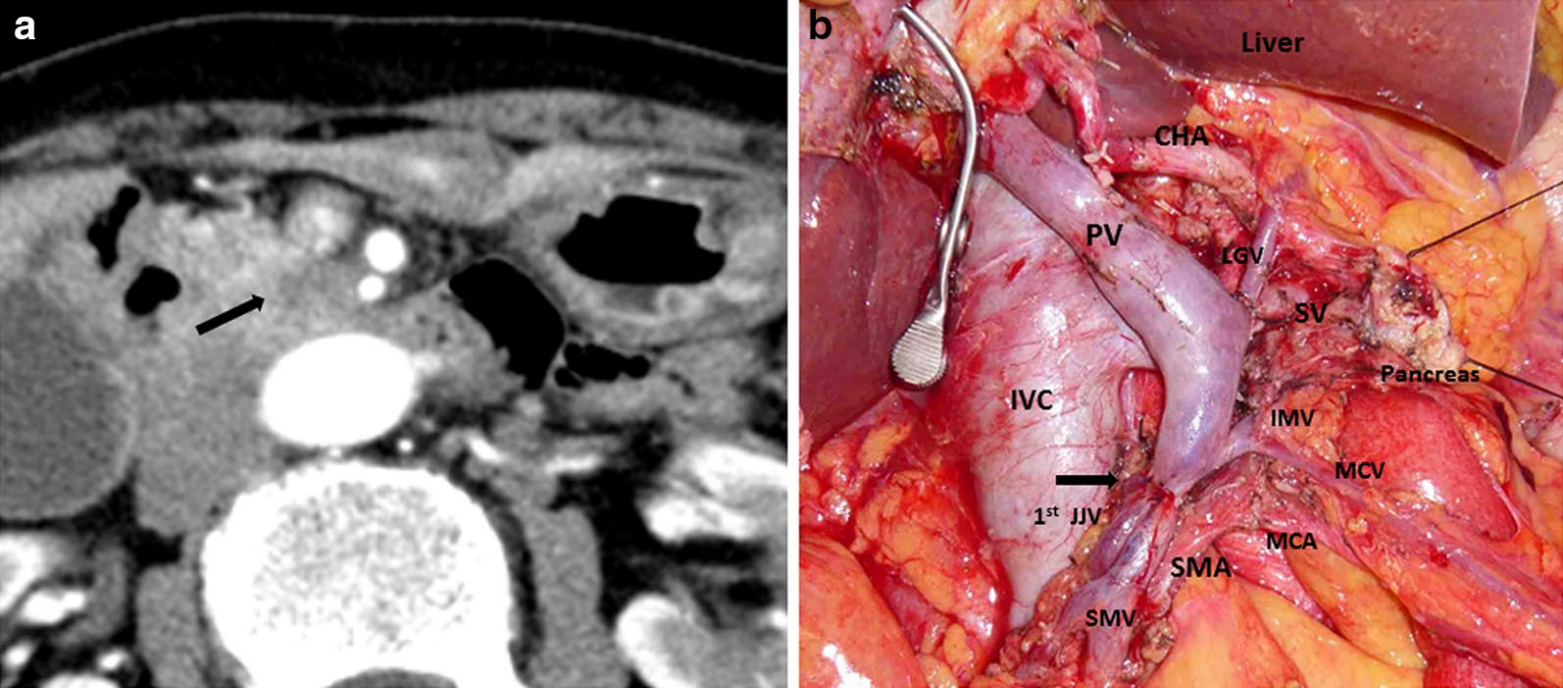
a Contrast-enhanced computed tomography (CECT) scan showing a hypovascular tumor abutting the superior mesenteric artery (SMA) and involving < 180° of the circumference of the artery without encasement. b Intraoperative photographs. The black arrowhead shows the anastomosis of the superior mesenteric vein with the 1^st^ jejunal vein. SMA: superior mesenteric artery. SMV: superior mesenteric vein. 1st JJV: 1st jejunal vein. PV: portal vein. MCA: middle colic artery. MCV: middle colic vein. IMV: inferior mesenteric vein. SV: splenic vein. LGV: left gastric vein. IVC: inferior vena cava
